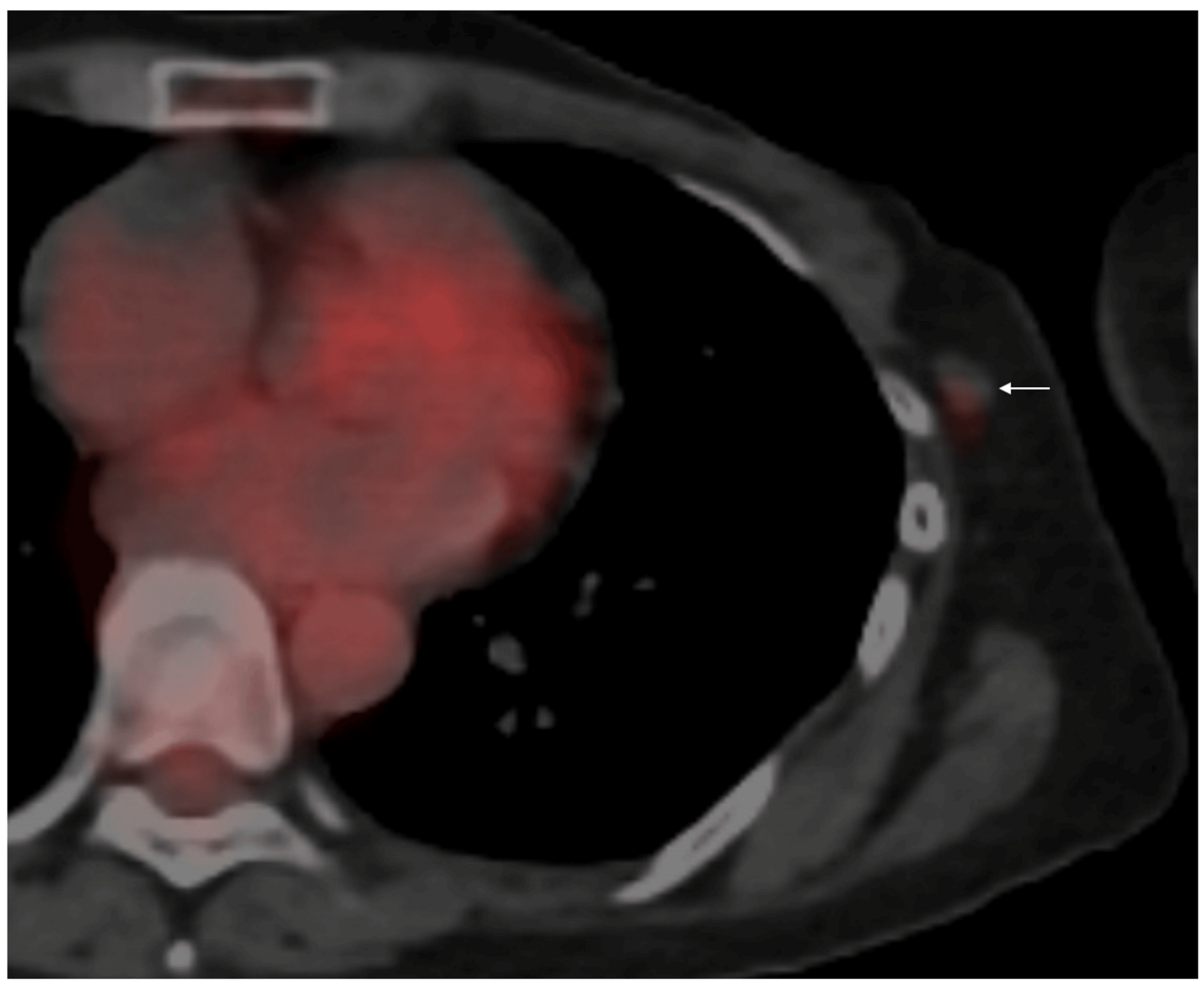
Positron emission tomography (PET)/computed tomography (CT) findings PET/CT showed no avid radio tracer uptake (arrow) in the axillary mass, strongly suggesting that malignant cells, even if present, were very sparsely present in the target lesion.
